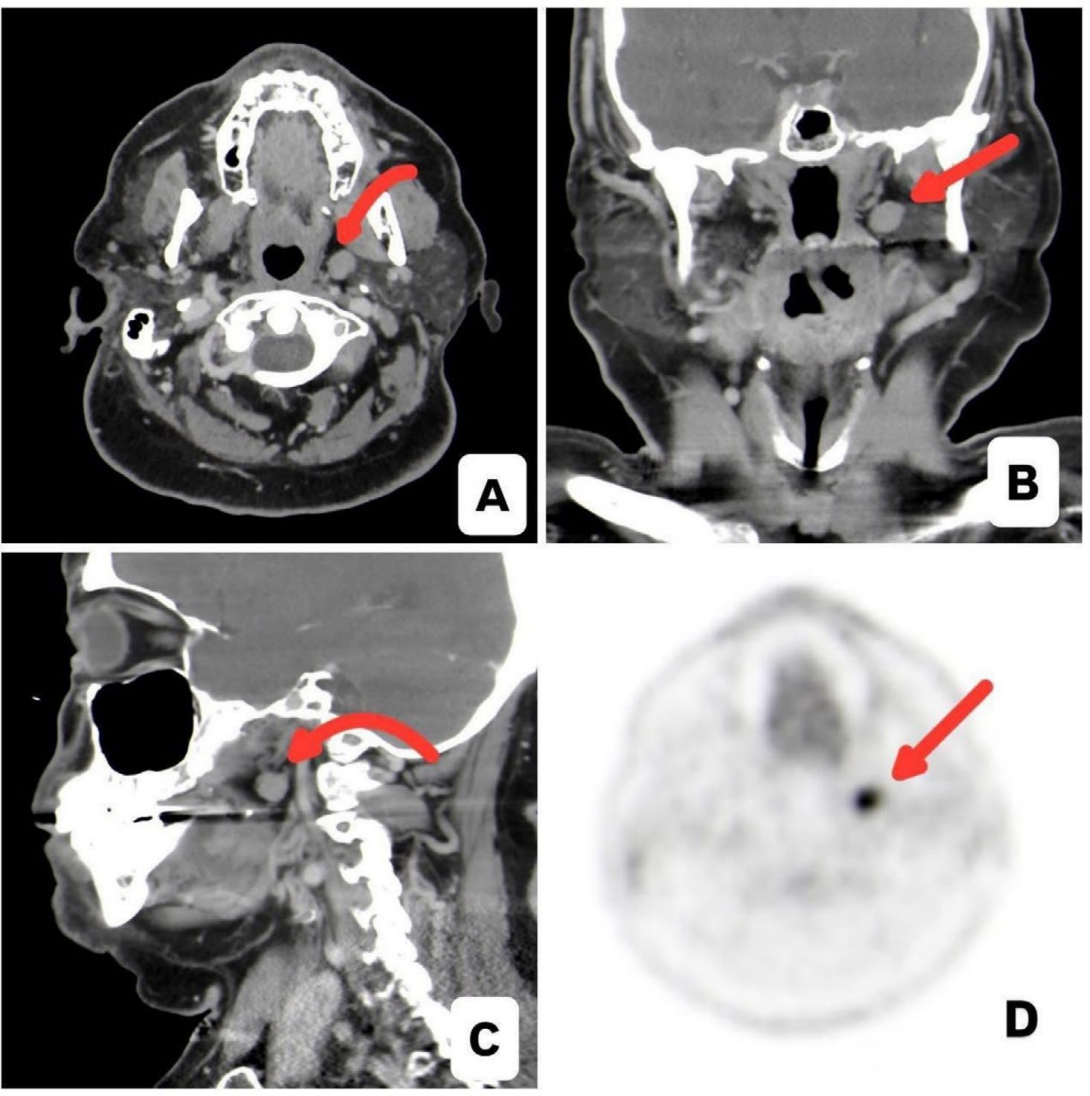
(A–C) Contrast‐enhanced CT images of the neck (A: Axial, B: Coronal, C: Sagittal) demonstrate a small, well‐defined left parapharyngeal nodular lesion, initially suspected to represent an abnormal lymph node. The lesion measures approximately 0.9 cm and shows soft‐tissue attenuation without aggressive features, surrounding fat stranding, or mass effect on adjacent structures. (D) Fused PET/CT image reveals marked FDG avidity within the previously suspected lymph node (SUVmax 9.7), significantly above physiologic background activity in the region.
